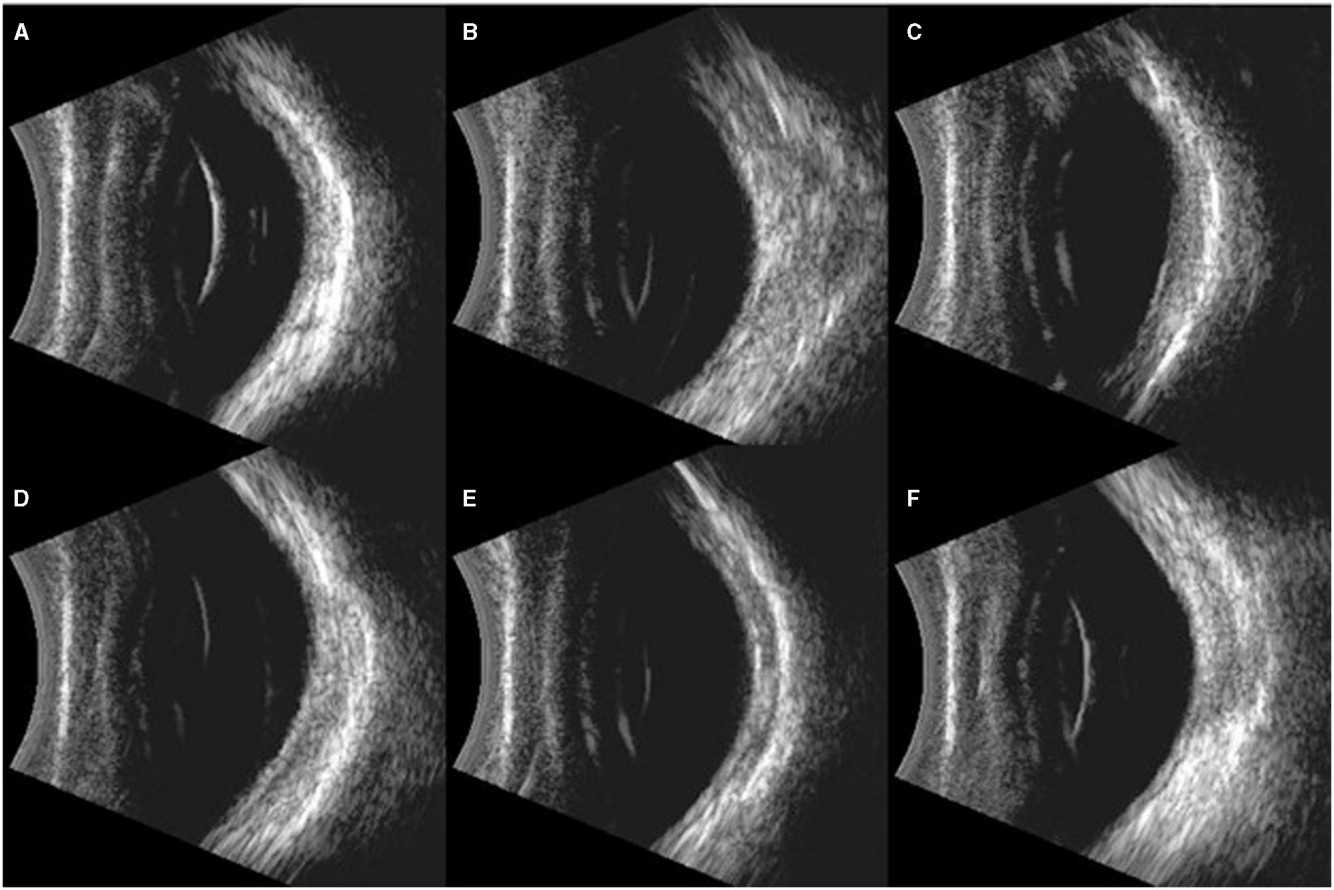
Typical images of B ultrasound scan on the eyes of rabbits. (A): Blank control group: Retina was well-attached with clear vitreous. (B–F): Eyes exposed to 500, 1,000, 1,500, 2,000, and 5,000 Kpa: Retina was well attached, and no vitreous lumpy echo was detected.
